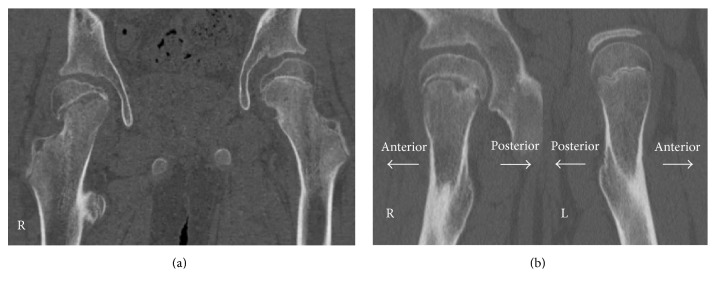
Reconstruction coronal (a) and sagittal (b) CT images of the right hip showing lateral displacement of the epiphysis.
